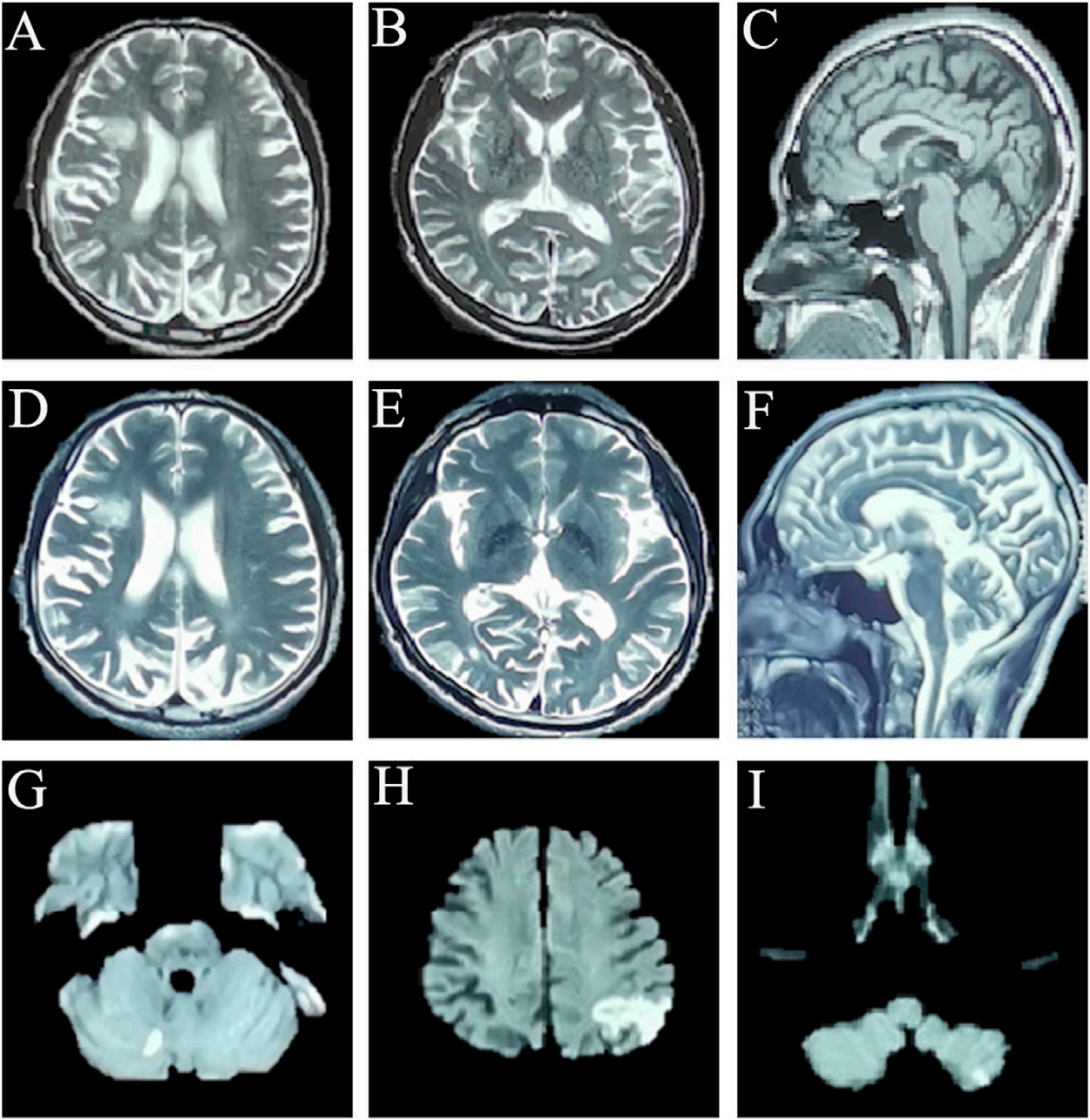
Cranial magnetic resonance imaging of this patient: (A–C) cross-sectional sequences of T2-weighted image (T2WI) (A,B) and sagittal image of T1-weighted image (T1WI) (C) (17 December 2019) showed the encephalomalacia in the right basal ganglia, frontal lobe and cerebral atrophy. (D–F) Cross-sectional sequences and sagittal images of T2WI (31 August 2021) showed that encephalomalacia foci had expanded to bilateral frontal lobe, right insula, and right temporo-occipital junction; brain atrophy is more worse than before. (G) Diffusion-weighted imaging (DWI) sequence showed punctate hyperintensity singals on the right cerebellum (22 September 2021). (H,I) DWI sequences showed abnormally high signal intensity on left parietal-occipital junction, and the left cerebellum (10 October 2021).
